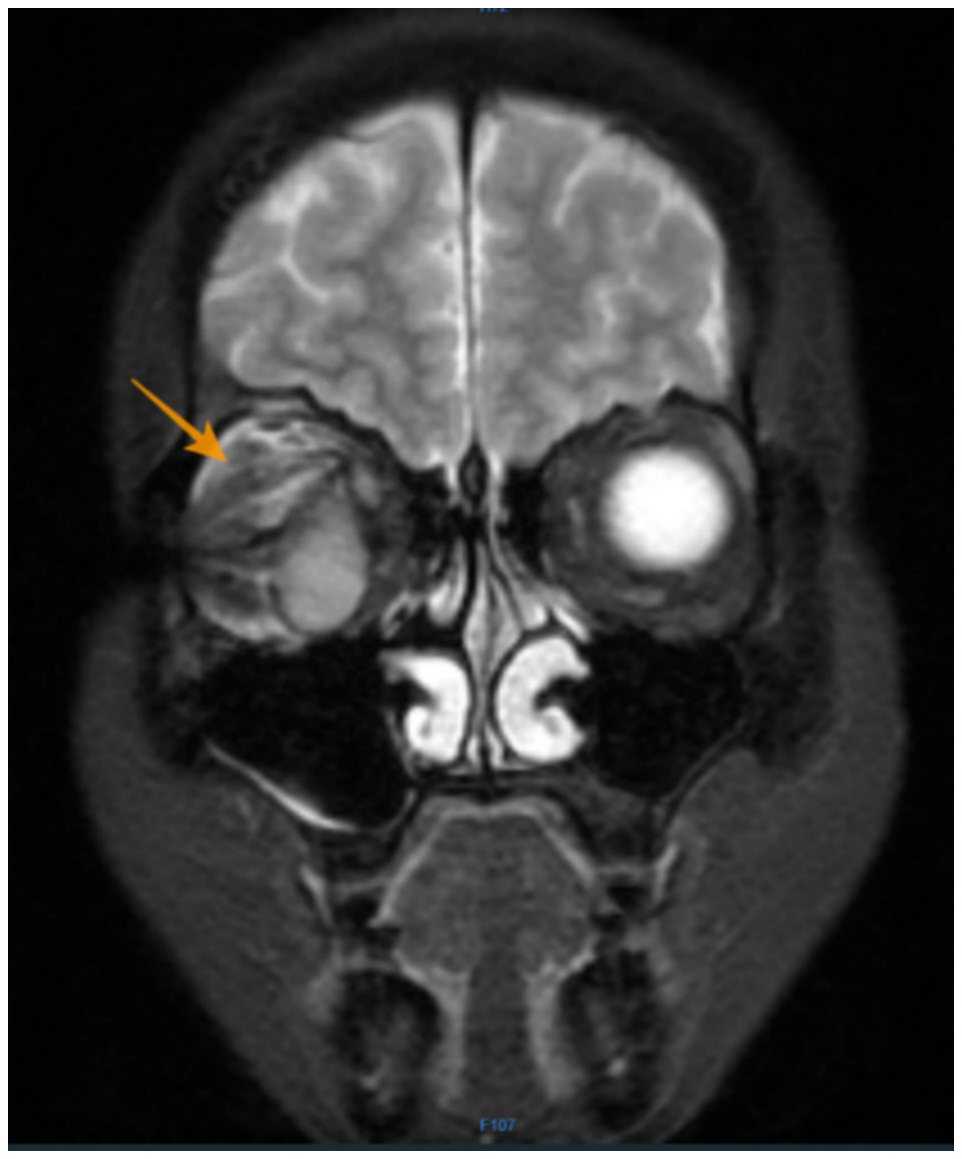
Orbital MRI reexamination on July, 2024: structural disorganization of the soft tissues in the periorbital space, with patchy mixed high signal intensity on T2WI and irregular enhancement. The lesion demonstrates ill-defined margins with the superior and lateral rectus muscles, measuring approximately 1.5 × 0.9 cm (the orange arrow).
